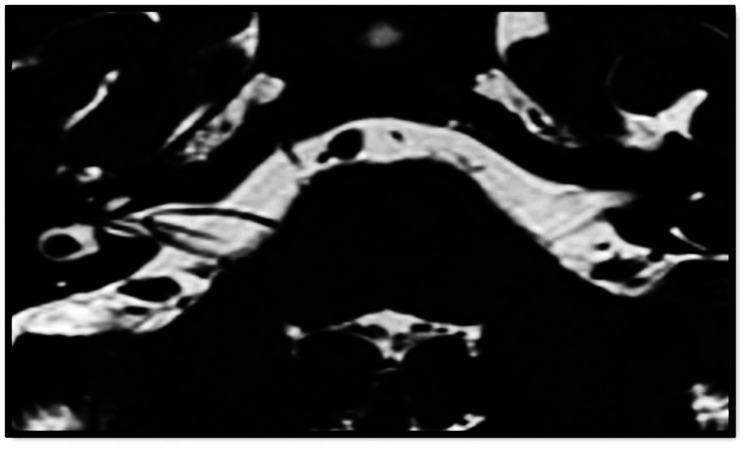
Chavda type II vascular loop. Axial high-resolution T2-weighted 3D-CISS sequence demonstrates the right AICA loop extending into the IAC (<50%). CISS = constructive interference in steady state; AICA = anterior inferior cerebellar artery; IAC = internal auditory canal
